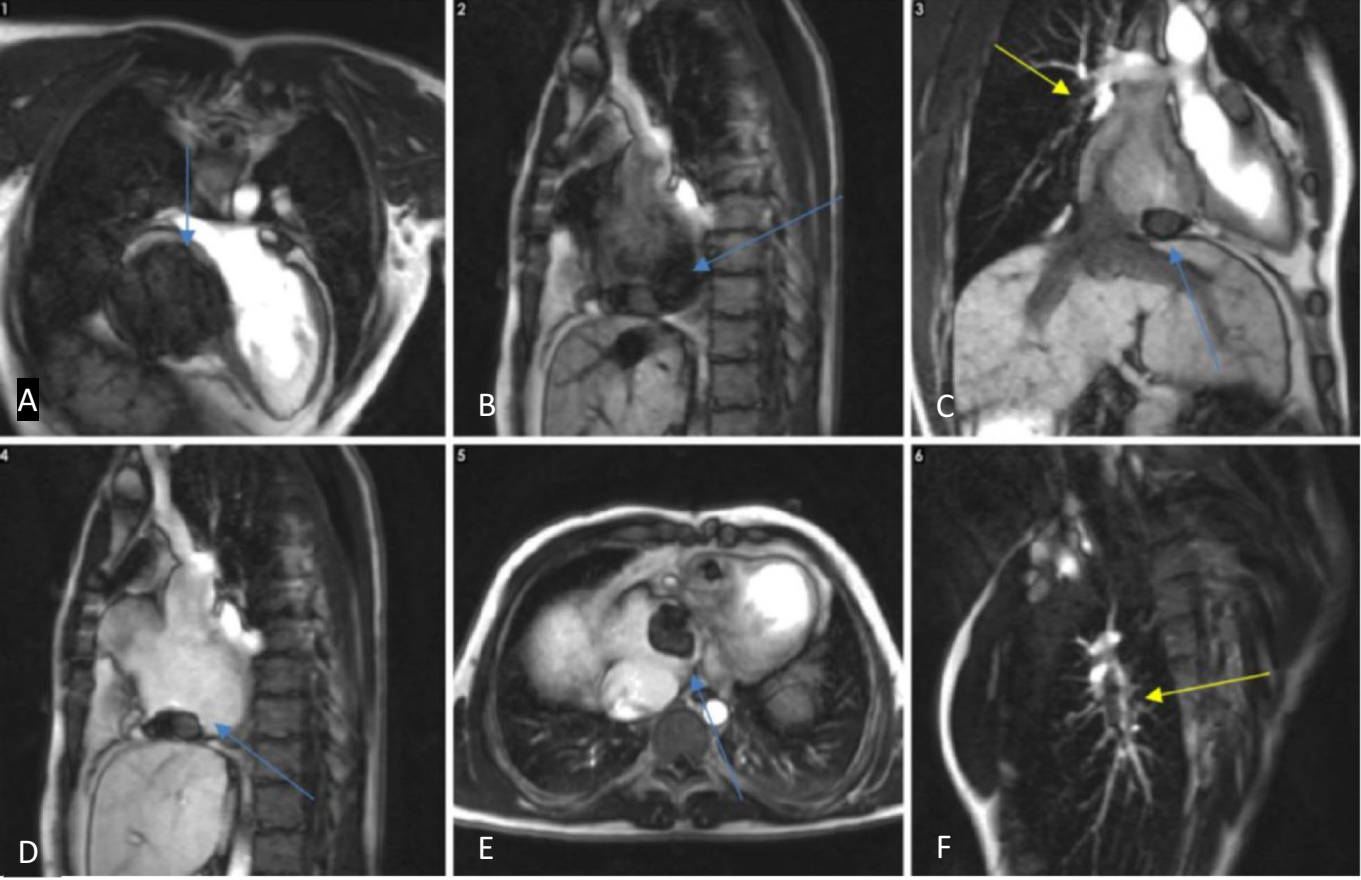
Horizontal long-axis (A), sagittal (B, D), coronal (C), axial (E) and right pulmonary artery cross-cut views (F) of a cardiovascular MRI study in an atriopulmonary Fontan circulation performed for congenital tricuspid atresia. There are filling defects in keeping with thrombi in the right atrium (blue arrows) and in the right pulmonary artery (yellow arrows) on inversion recovery early-phase gadolinium sequences. Note that thrombus appears as very hypointense. Re-used with permission from Fontan circulation in an adult: A guide for the radiologist. Arzanauskaite M, Nyktari E, Voges I. ESTI-ESCR 2018/P-0103
